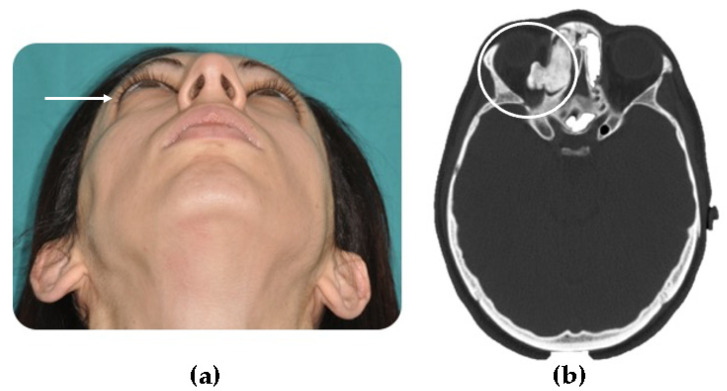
(a) A young patient presenting right exophthalmos (white arrow) due to an ethmoid osteoma with orbital invasion; (b) CT scan shows an extensive right ethmoid osteoma which invades the orbital cavity (circled in CT scan).
